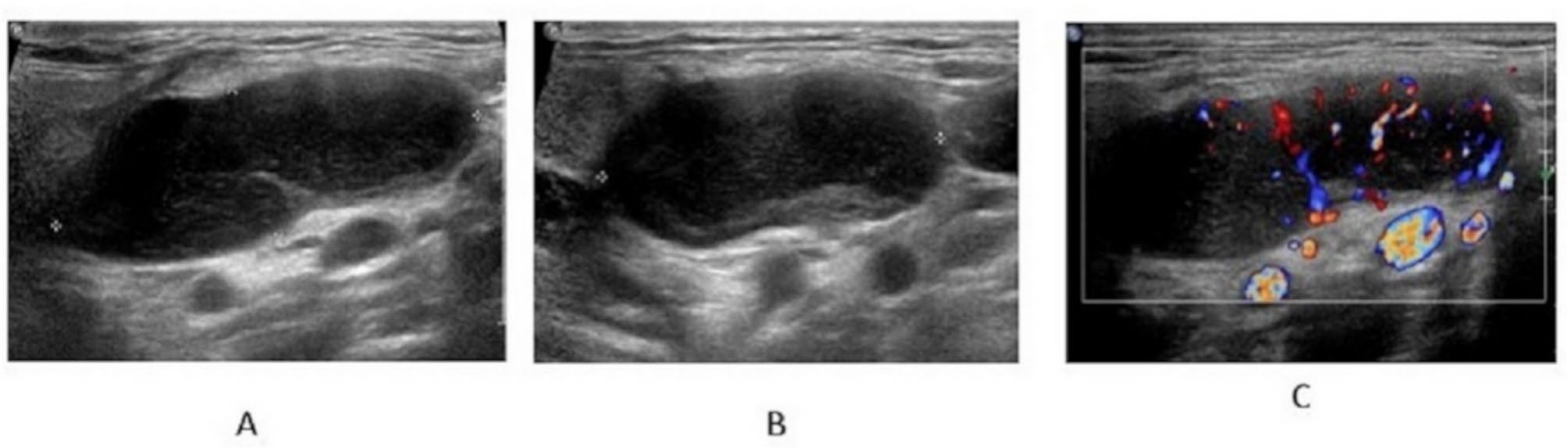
US images from a 36-year-old man with a palpable mass in the left cervical region. The pathological diagnosis of the enlarged LN was the hyaline vascular type (A-B) The enlarged cervical lymph node grayscale US. The size of the lymph nodes was 4.7 × 3.4 × 1.7 cm. The LN showed a thickened cortex. Short linear hyperechoic areas are visible within the LN. (C) The color Doppler image. The LN showed abundant hilar color flow US, ultrasound; LN, lymph node; cm, centimeter
